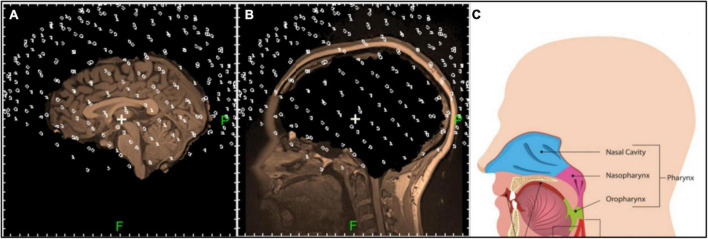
Sagittal tomographic sections of the «brain» (A) and «non-brain» (B) areas of the head after the annotation of initial MRI. Positions of the sensors are denoted with white rings. Anatomy of the possible strong non-brain sources is shown in panel (C).
